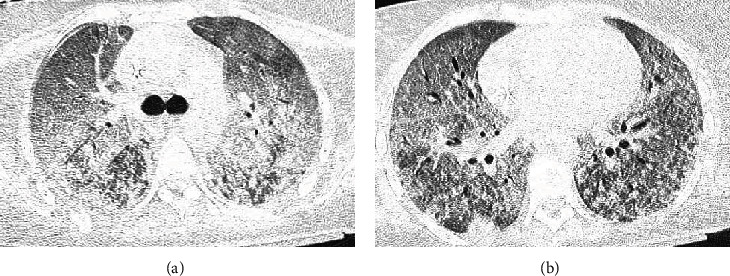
(a, b) CT images showing the dramatic worsening of the lung injury leading to the beginning of venovenous ECMO support to improve both oxygenation and CO_2_ removal.
